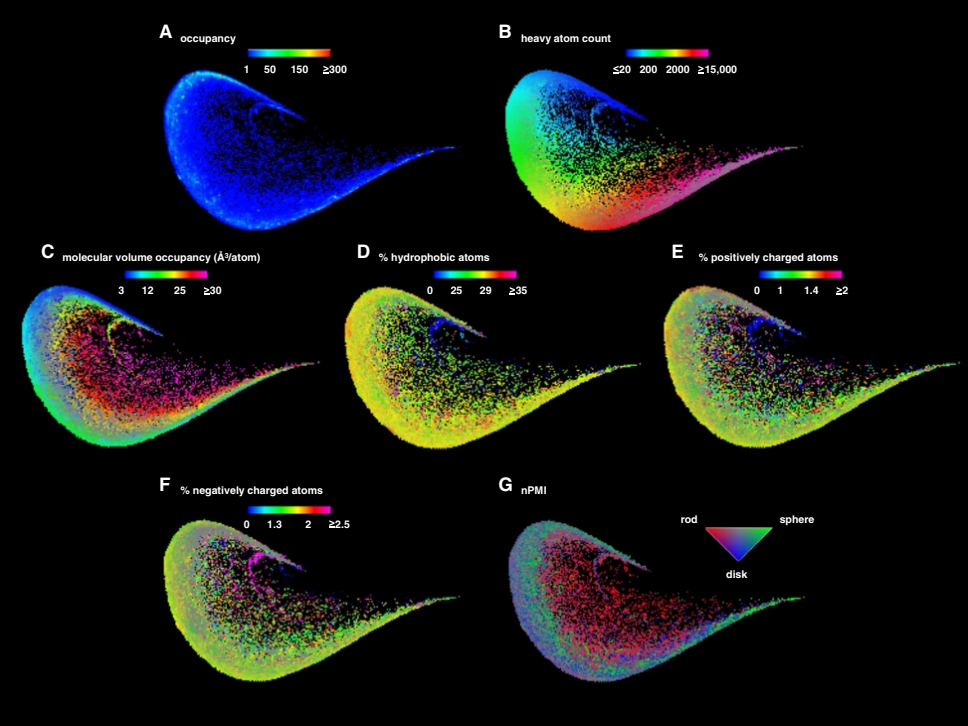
PDB-maps of 3DP-similarity space color-coded by (a) occupancy, (b) heavy atom count, (c) molecular volume occupancy (mvo), (d) percentage of hydrophobic atoms, (e) percentage of positively charged atoms, (f) percentage of negatively charged atoms. The color-coding is from *blue* (lowest values) to *magenta* (highest values). (g) PDB-map color-coded by nPMI values. The rod-like structures are *red* color; the spherical structures are *green* color; the disc-like structures are *blue* color. The maps were computed from 91,223 X-ray structures from the PDB downloaded in September 2014, considering in each case the biological assembly as defined by the author
